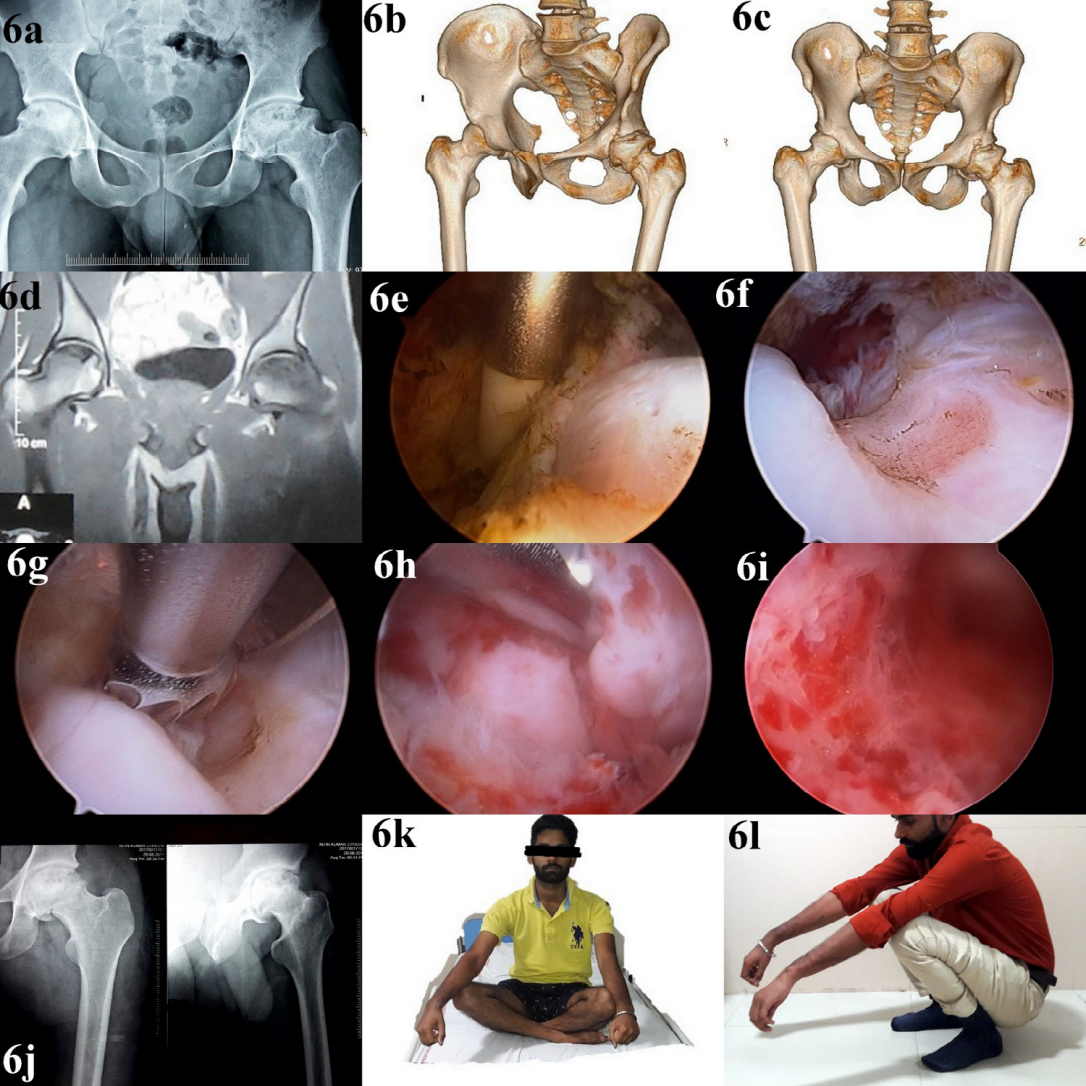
Follow-up of a 33-year-old male presenting with symptoms of hip impingement after alcohol-induced ONFH; panel a: preoperative radiograph showing cam deformity in right femoral head; panels b and c: preoperative CT scan showing extent and location of cam deformity in the femoral head; panel d: MRI of the hip joint done 1.8 years before the surgery confirming ONFH bilaterally; panel e: intraoperative image showing arthroscopy of the peripheral compartment of the hip joint using outside-in capsulotomy; panel f: intraoperative image of hip arthroscopy showing identification of the cam deformity; panel g: intraoperative image of hip arthroscopy showing resection of the cam deformity; panel h: intraoperative image of hip arthroscopy showing cam deformity in the anterolateral part of femoral neck; panel i: intraoperative image of hip arthroscopy after removal of the deformity; panel j: post-operative radiograph after removal of cam deformity; panels k and l: post-operative follow-up images showing restoration of hip range of motion.
